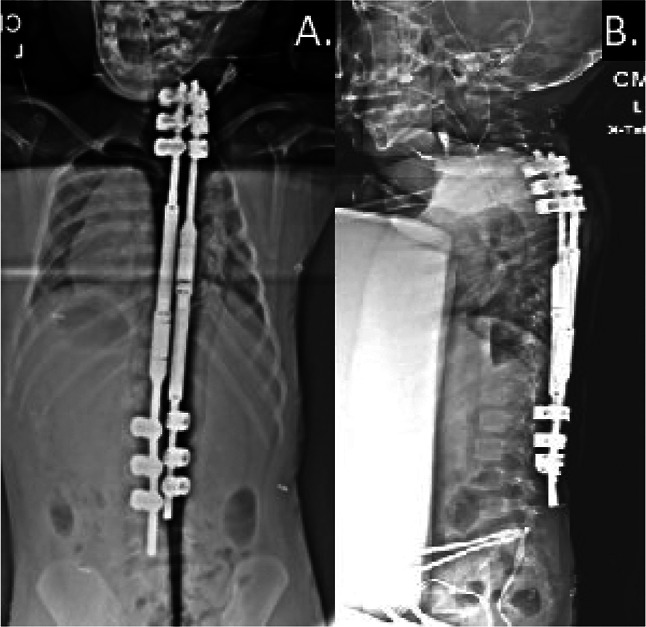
Postoperative radiographic images demonstrating MAGEC rods immediately after placement of the rods. A, PA view demonstrating coronal alignment of the cervical, thoracic, and lumbar spine. B, Lateral view demonstrating sagittal alignment of the cervical, thoracic, and lumbar spine.
